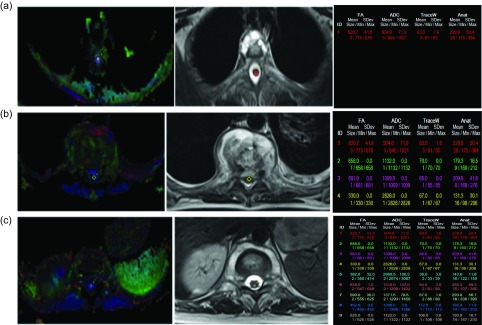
(a) Left: circular ROI drawn for calculation of FA and MD/ADC values on diffusion FA map above the site of lesion (SOL). Middle: corresponding T2 axial MRI image shows no disease above the SOL. Right: once circular ROIs were drawn, FA and MD values were generated which were recorded. Three such ROIs were drawn above the SOL. (b) Left: circular ROI drawn at the SOL. Middle: corresponding axial MRI image shows disease and compression on cord. Right: FA and MD values generated in the same color as ROI. Note: The first three values are for above the SOL. (c) Left: circular ROI drawn below the SOL. Middle: the corresponding axial MRI image shows no disease. Right: FA and MD values generated. Values (1–3) – above SOL (4–6) – at SOL (7–9) – below SOL.
